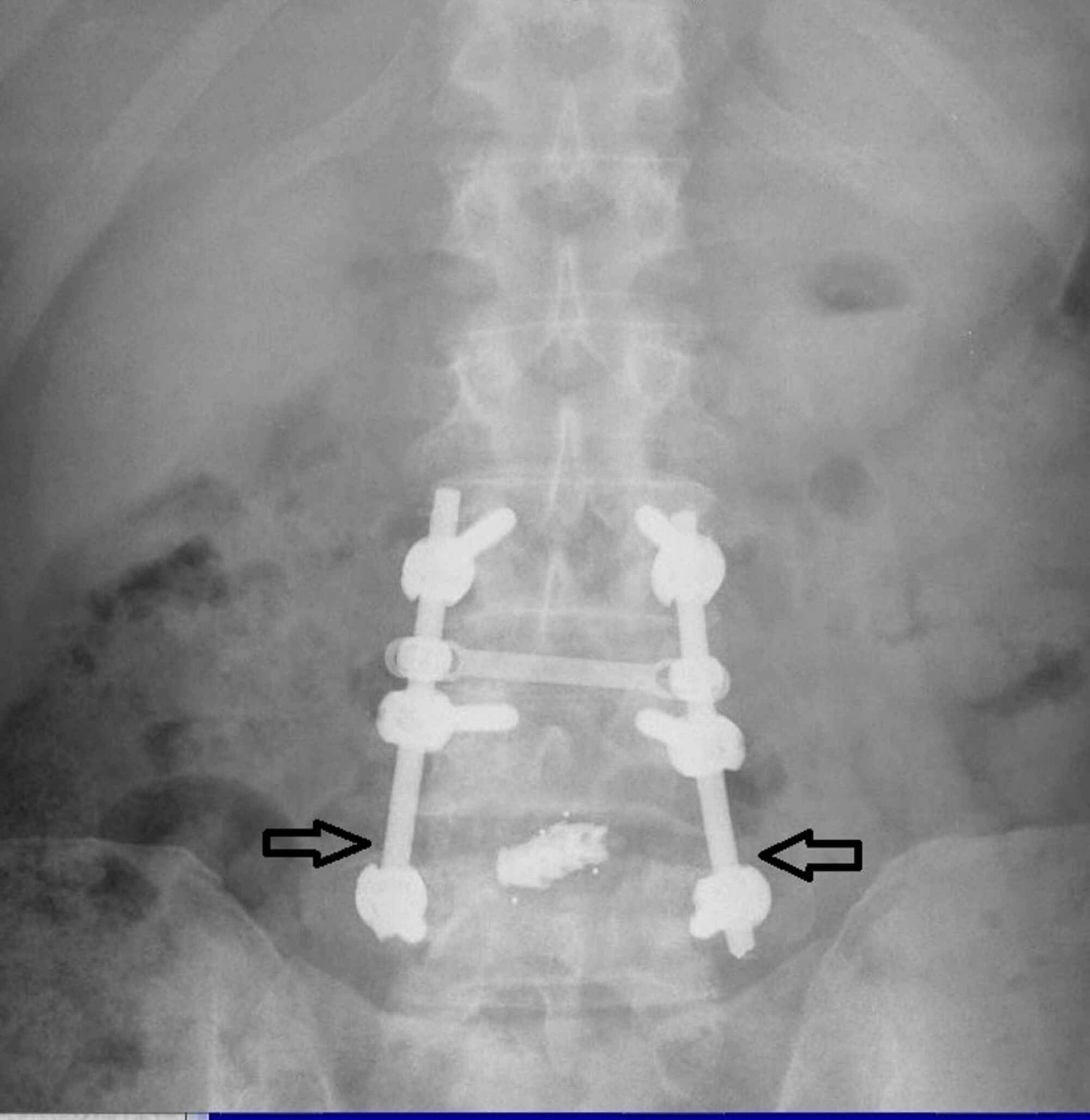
Postoperative (three months follow up) standing anteroposterior roentgenogram of the lumbar spine. The black arrows show the level of the interbody fusion.
